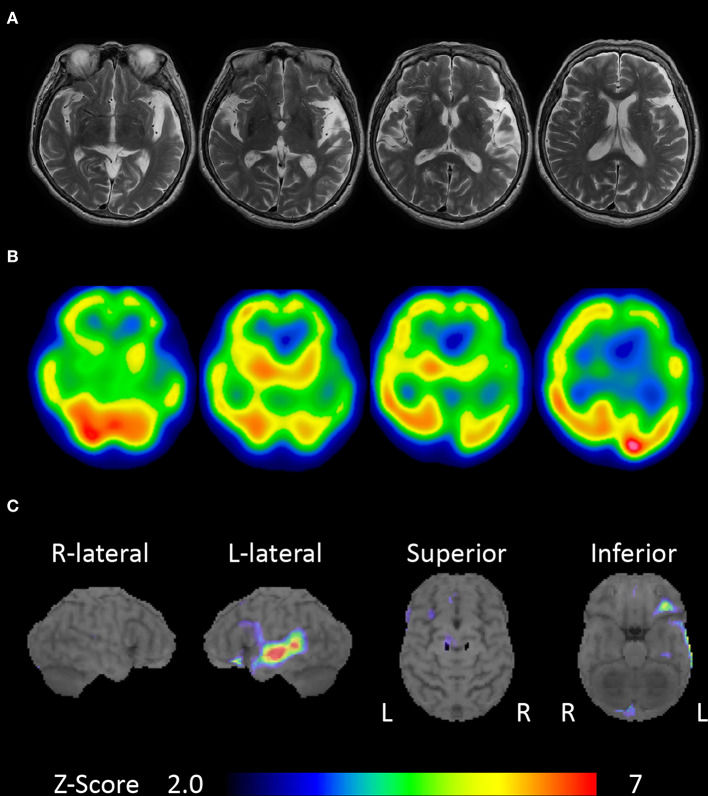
Brain magnetic resonance imaging and single-photon emission computed tomography (SPECT). (A) Brain magnetic resonance imaging showing left-sided predominant atrophy of the perisylvian area. (B)
